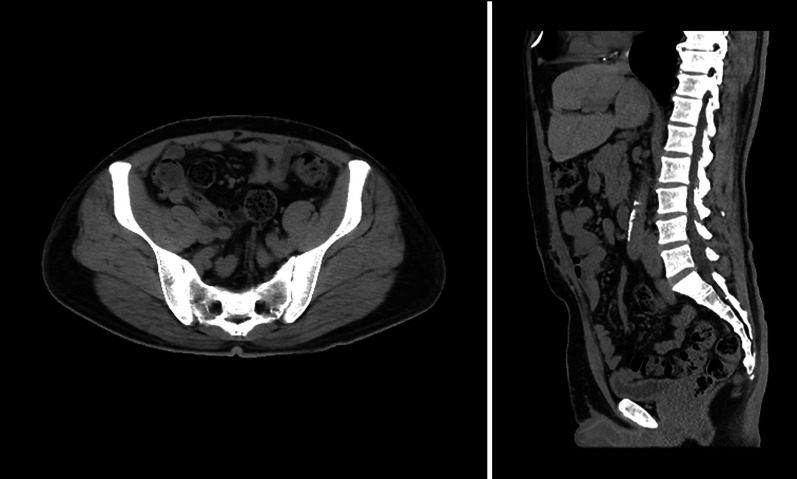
CT scan images at 4 months follow-up
